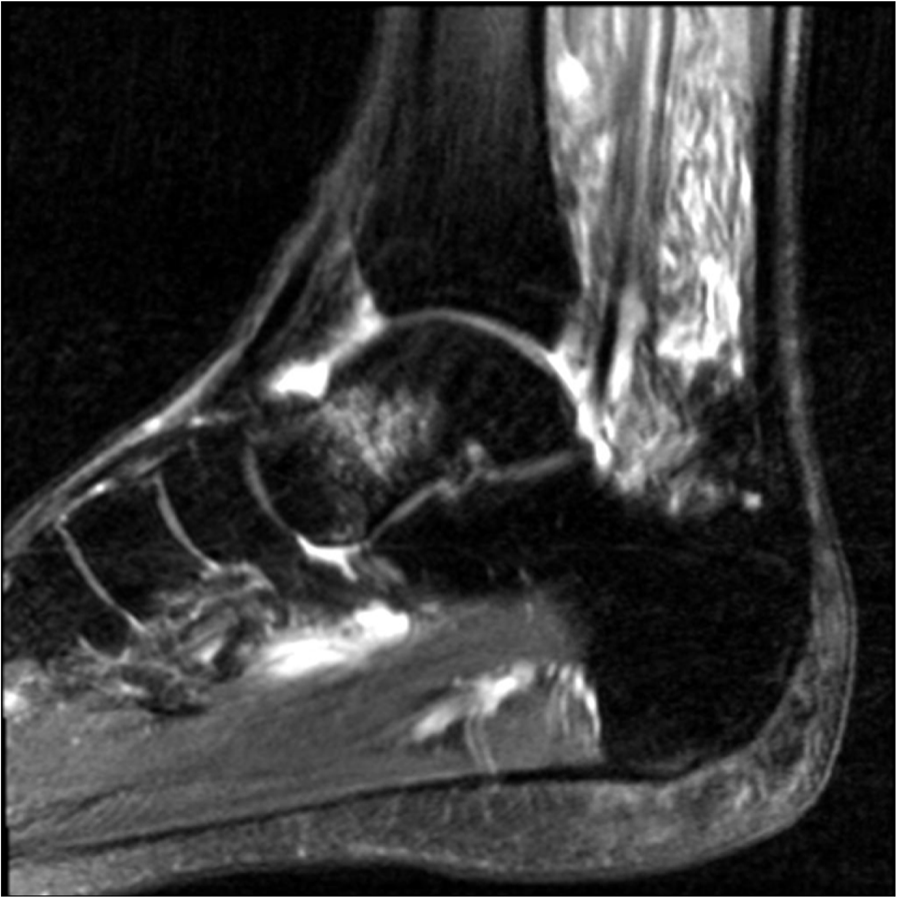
T2 sagittal MRI of the ankle demonstrating grade II stress fracture/stress reaction of the talar neck
